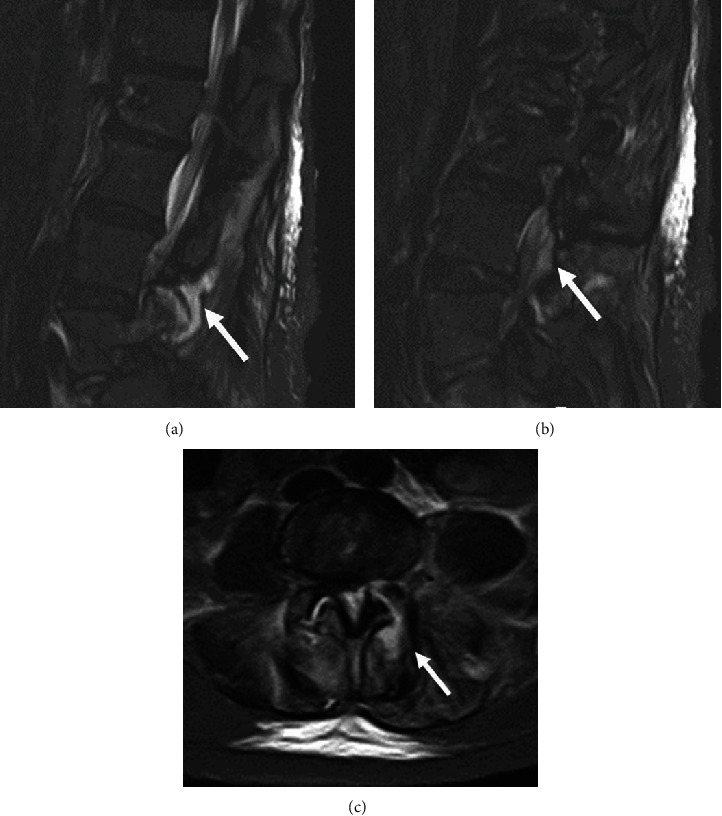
MRI images on the 5th day of hospitalization. Sagittal view (a and b) and axial view at L4/5 (c) of T2-STIR MRI showing an abscess from the facet joint to the paravertebral muscle (a, c) and epidural abscess (arrows).
